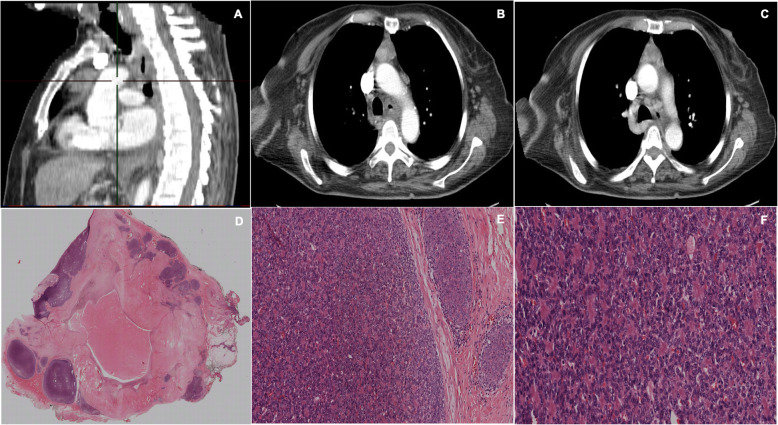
Chest CT scan. a, b, c coronal reconstruction. d, e, f. Sagittal scans. A well-defined mass of lobulated contours is observed, which compromises the anterior mediastinum towards the right hemithorax and extends in the cephalon-caudal direction towards the cardio phrenic recess on the ipsilateral side. It is homogenous, with soft tissue densify and it enhances with contrast medium. It is 13 × 10 × 15 cm, with few calcifications, and in close contact with the ascending aorta. There is a fatty plane between the superior vena cava and the mass
